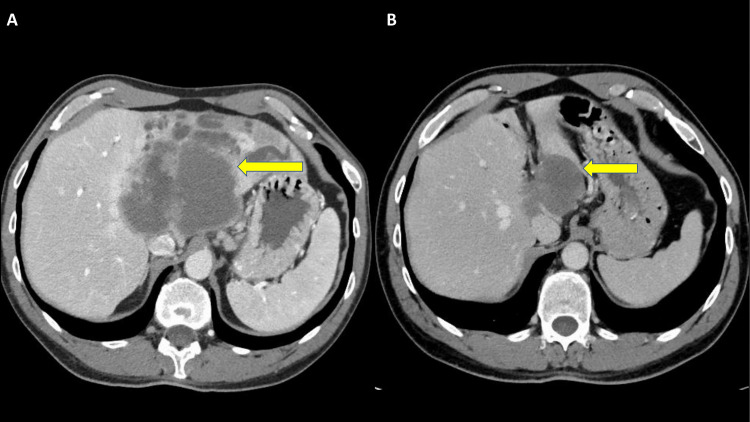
CT of the abdomen. CT scan of a large predominantly hypodense 10x8 cm mass in the central left hepatic lobe at presentation (A). Tumor size decreased to 6.2x4.3 cm after three months of starting immunotherapy (B).
